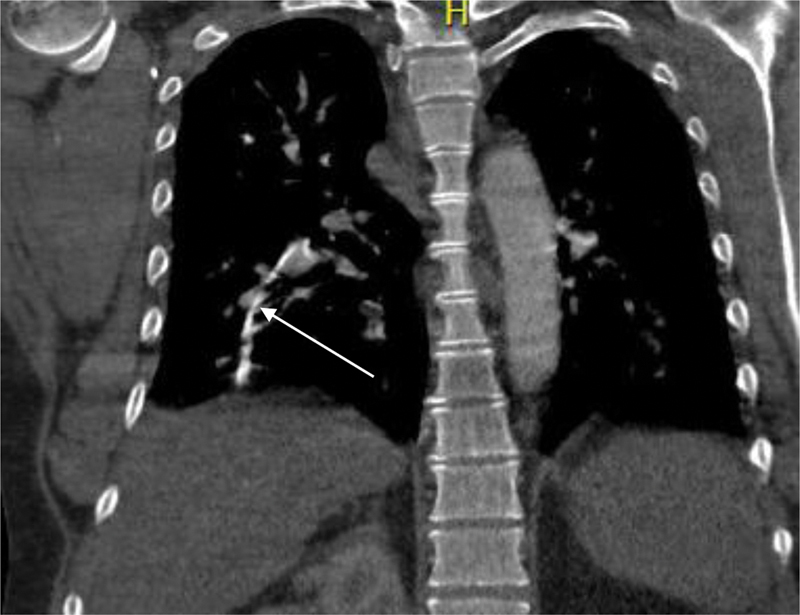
Coronal images of the CT-scan revealing the foreign body trapped in both pulmonal arteries, consistent with a CVC remnant with a possible false route in the right lung. CT, computed tomography; CVC, Central venous catheters.
